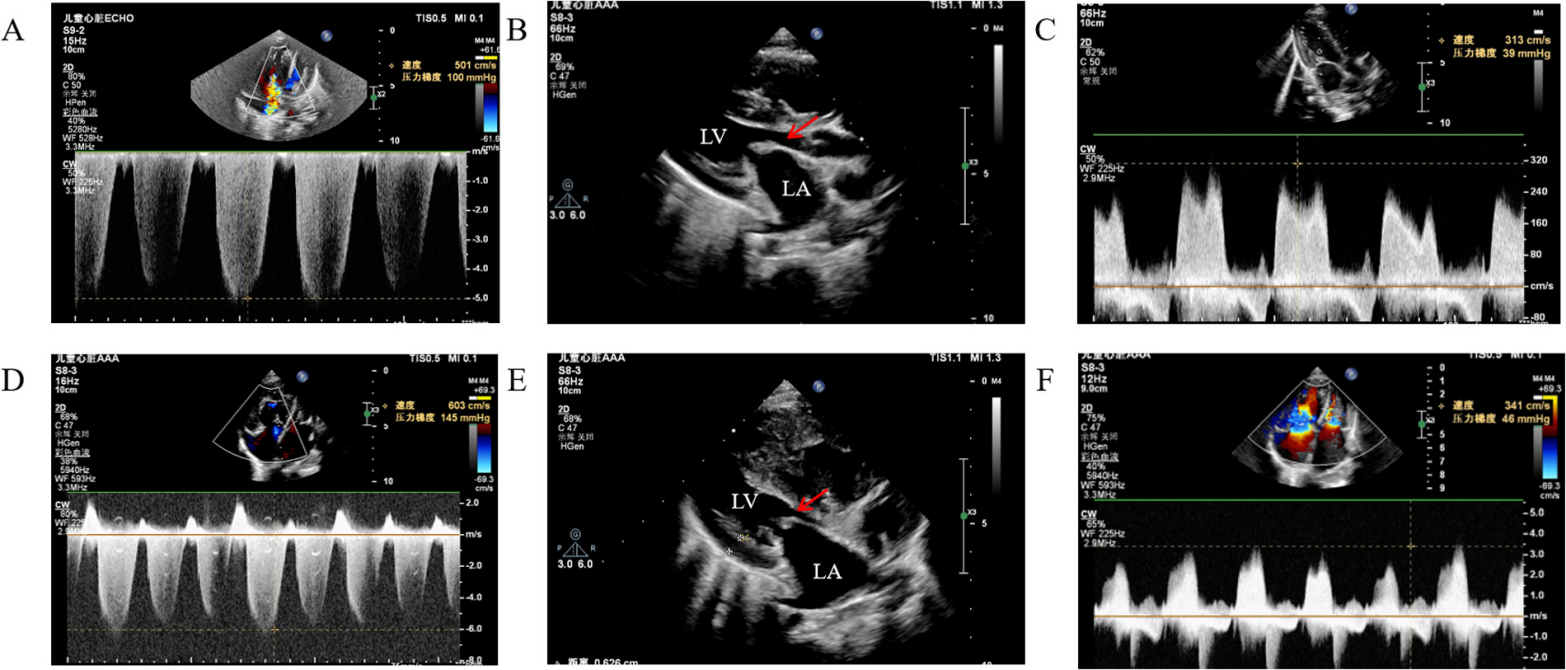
Cardiac ultrasound showed pulmonary artery pressure and progression of mitral stenosis. (A) pulmonary artery pressure 100 mmHg, (B) anterior mitral valve 3 mm, posterior valve 2.6 mm, orifice area 0.24 cm^2^, (C) mitral flow velocity 3.13 m/s, (D) pulmonary artery pressure 145 mmHg, (E) anterior mitral valve 3.7 mm, posterior valve 3.2 mm, orifice area 0.21 cm^2^, (F) mitral flow velocity 3.41 m/s. (A-C) results of October 2023 inspection, (D-F) results of December 2024 inspection.
